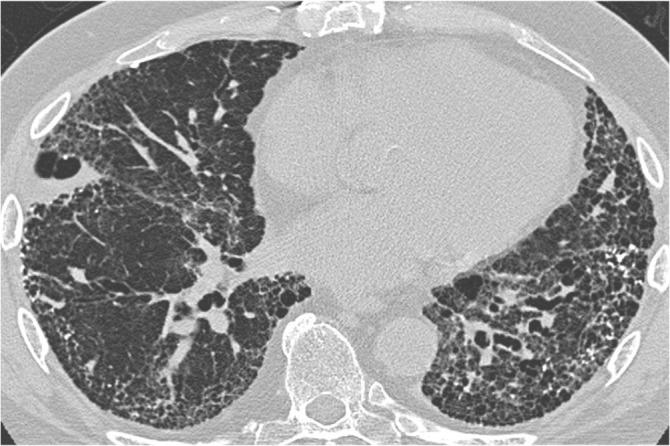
High resolution computed tomography of the lungs in a patient with RA-ILD, demonstrating changes of usual interstitial pneumonia with bibasal fibrosis and early honeycombing.
